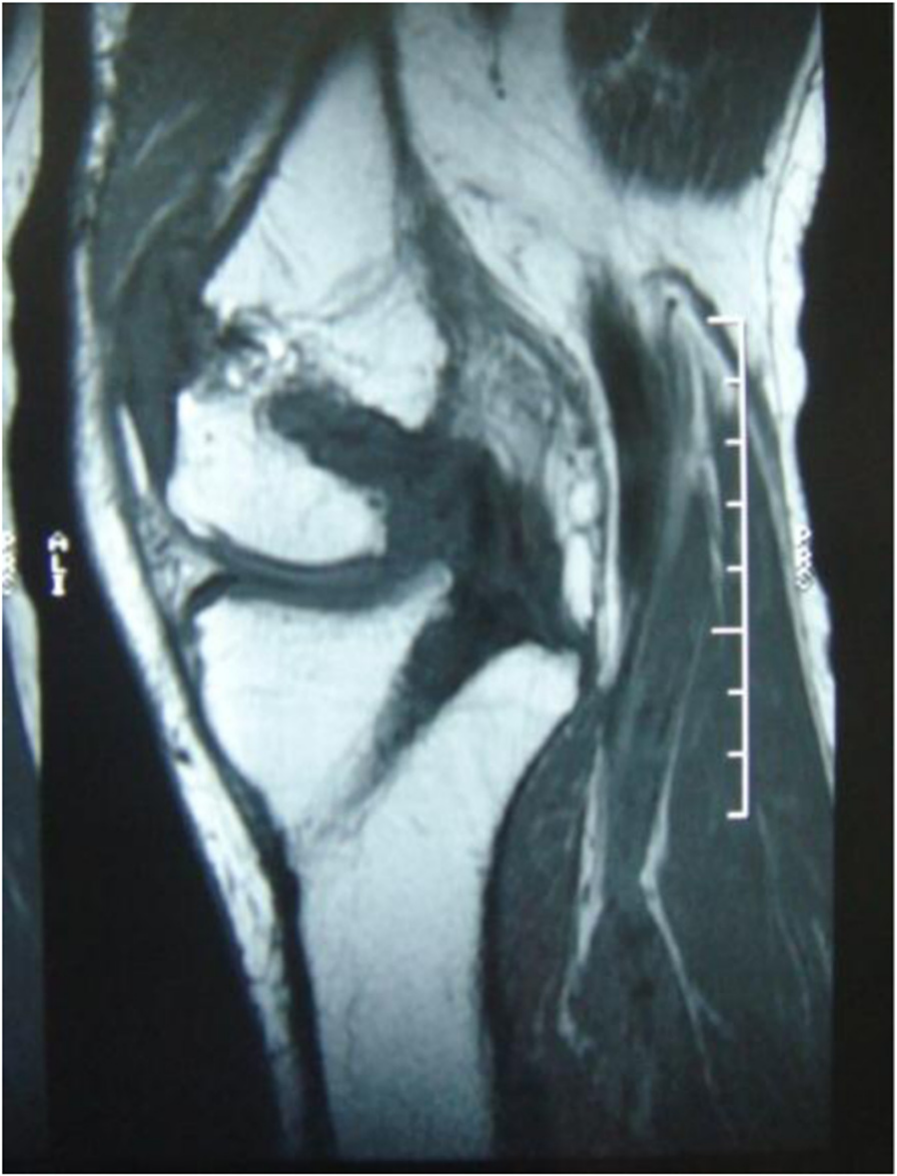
MRI of left knee 5 years after operation showing the satisfactory morphology of the reconstructed PCL and no signs of recurrence.
